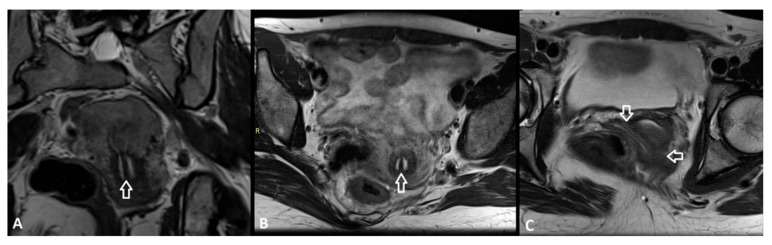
27-year-old female with complete septate uterus, cervix and vagina. Axial T2-weighted MR images at the level of the uterine corpus (A), cervix (B) and vagina (C). The septum is demonstrated with white arrows. The vaginal septum is longitudinal and non-obstructing. This Müllerian duct anomaly is described in the ASRM classification as a ‘complete septate uterus with septate cervix and longitudinal vaginal septum’. In the ESHRE/ESGE classification, this is a U2b C1 V1. The AFS classification only mentions complete septate uterus.
